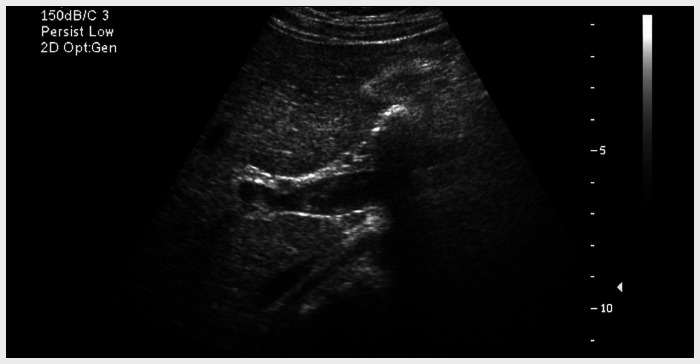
Abdominal ultrasound on eleventh postoperative day following laparoscopic splenectomy, revealing residual thrombus within the main portal vein with flow through the region, persistent thrombosis of the left portal vein, and patent right portal vein; the splenic vein was not seen.
